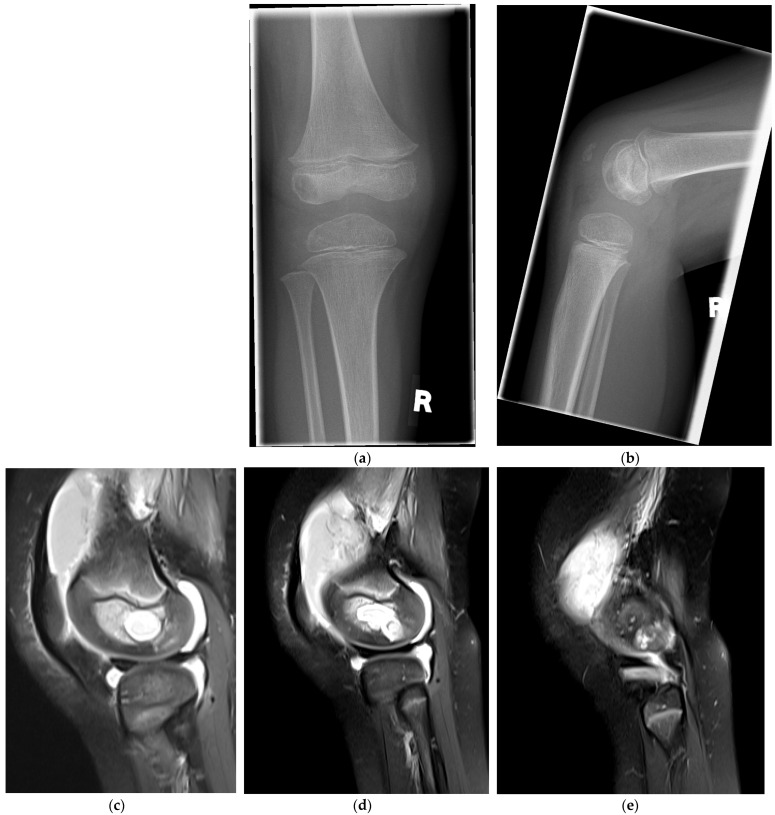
Plain radiographs of the right knee in anterior-posterior (a) and lateral (b) views after hospital admission, indicating the bone defect in the lateral femoral epiphysis reaching from the growth plate to the subchondral plate. Sagittal MRI (turbo-spin echo, fat saturation) demonstrating a centrally round bone defect with surrounding edema (c), in the lateral epiphysis, the defect reaching the subchondral bone (d) and penetrating into the knee through a cortical orifice (e).
